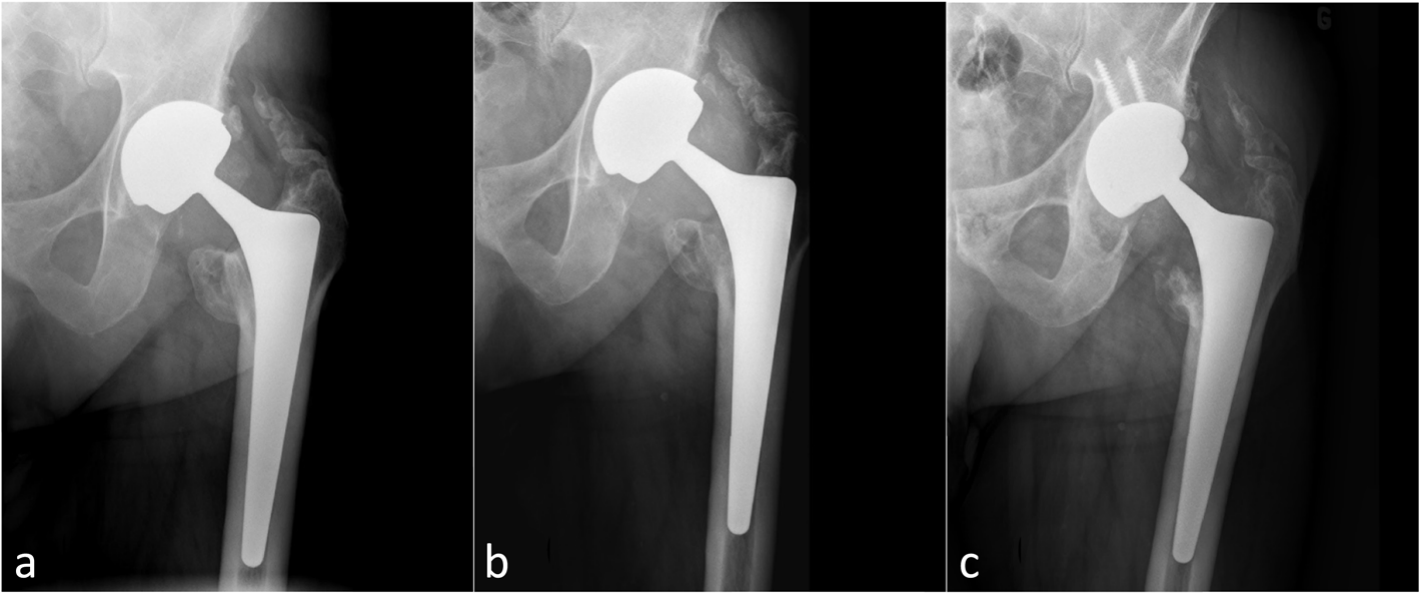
a A 63-year-old man with osteoarthritis underwent implantation of large diameter total hip arthroplasty implant. An AP radiograph of the patient’s left hip taken 1 month after surgery. b An AP radiograph of the patient’s right hip taken 11 years postoperatively shows proximal femoral lysis. c The patient underwent bipolar revision with a CoC implant
